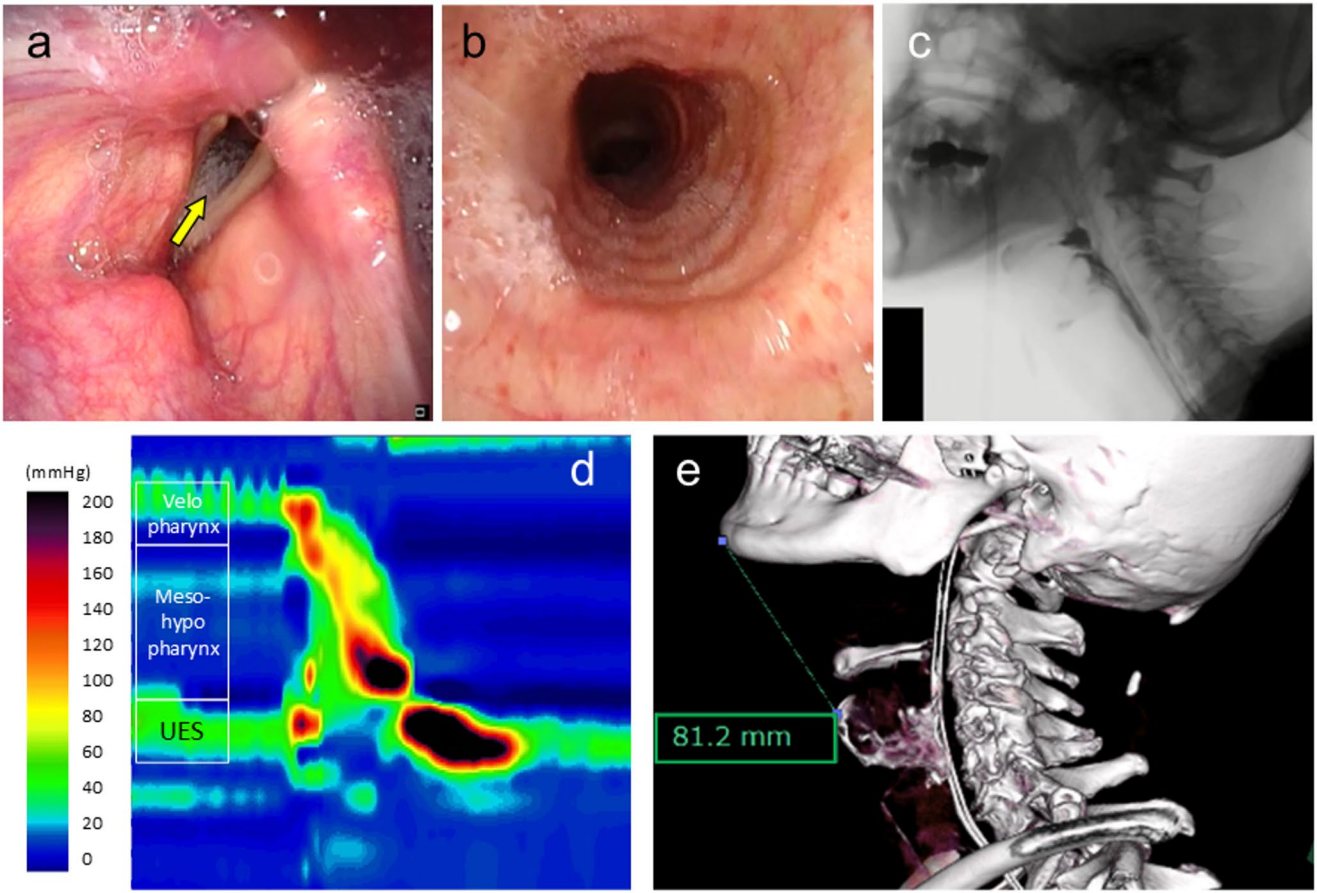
Preoperative examinations. (a, b) laryngoscopy revealed saliva pooling in the hypopharynx and entry of saliva into the trachea (yellow arrow). (c) videoflouroscopic swallowing study with 5 ml thickened contrast agent showed silent aspiration into the airway. (d) high-resolution manometry (HRM) tracing obtained during swallowing. (e) cervical computed tomography scan showed the preoperative thyromental distance of 81.2 mm. The potential extent of elevation and anteriorization of the larynx, as it approximates the mandible, can also be estimated during the planning process. UES: upper esophageal sphincter
